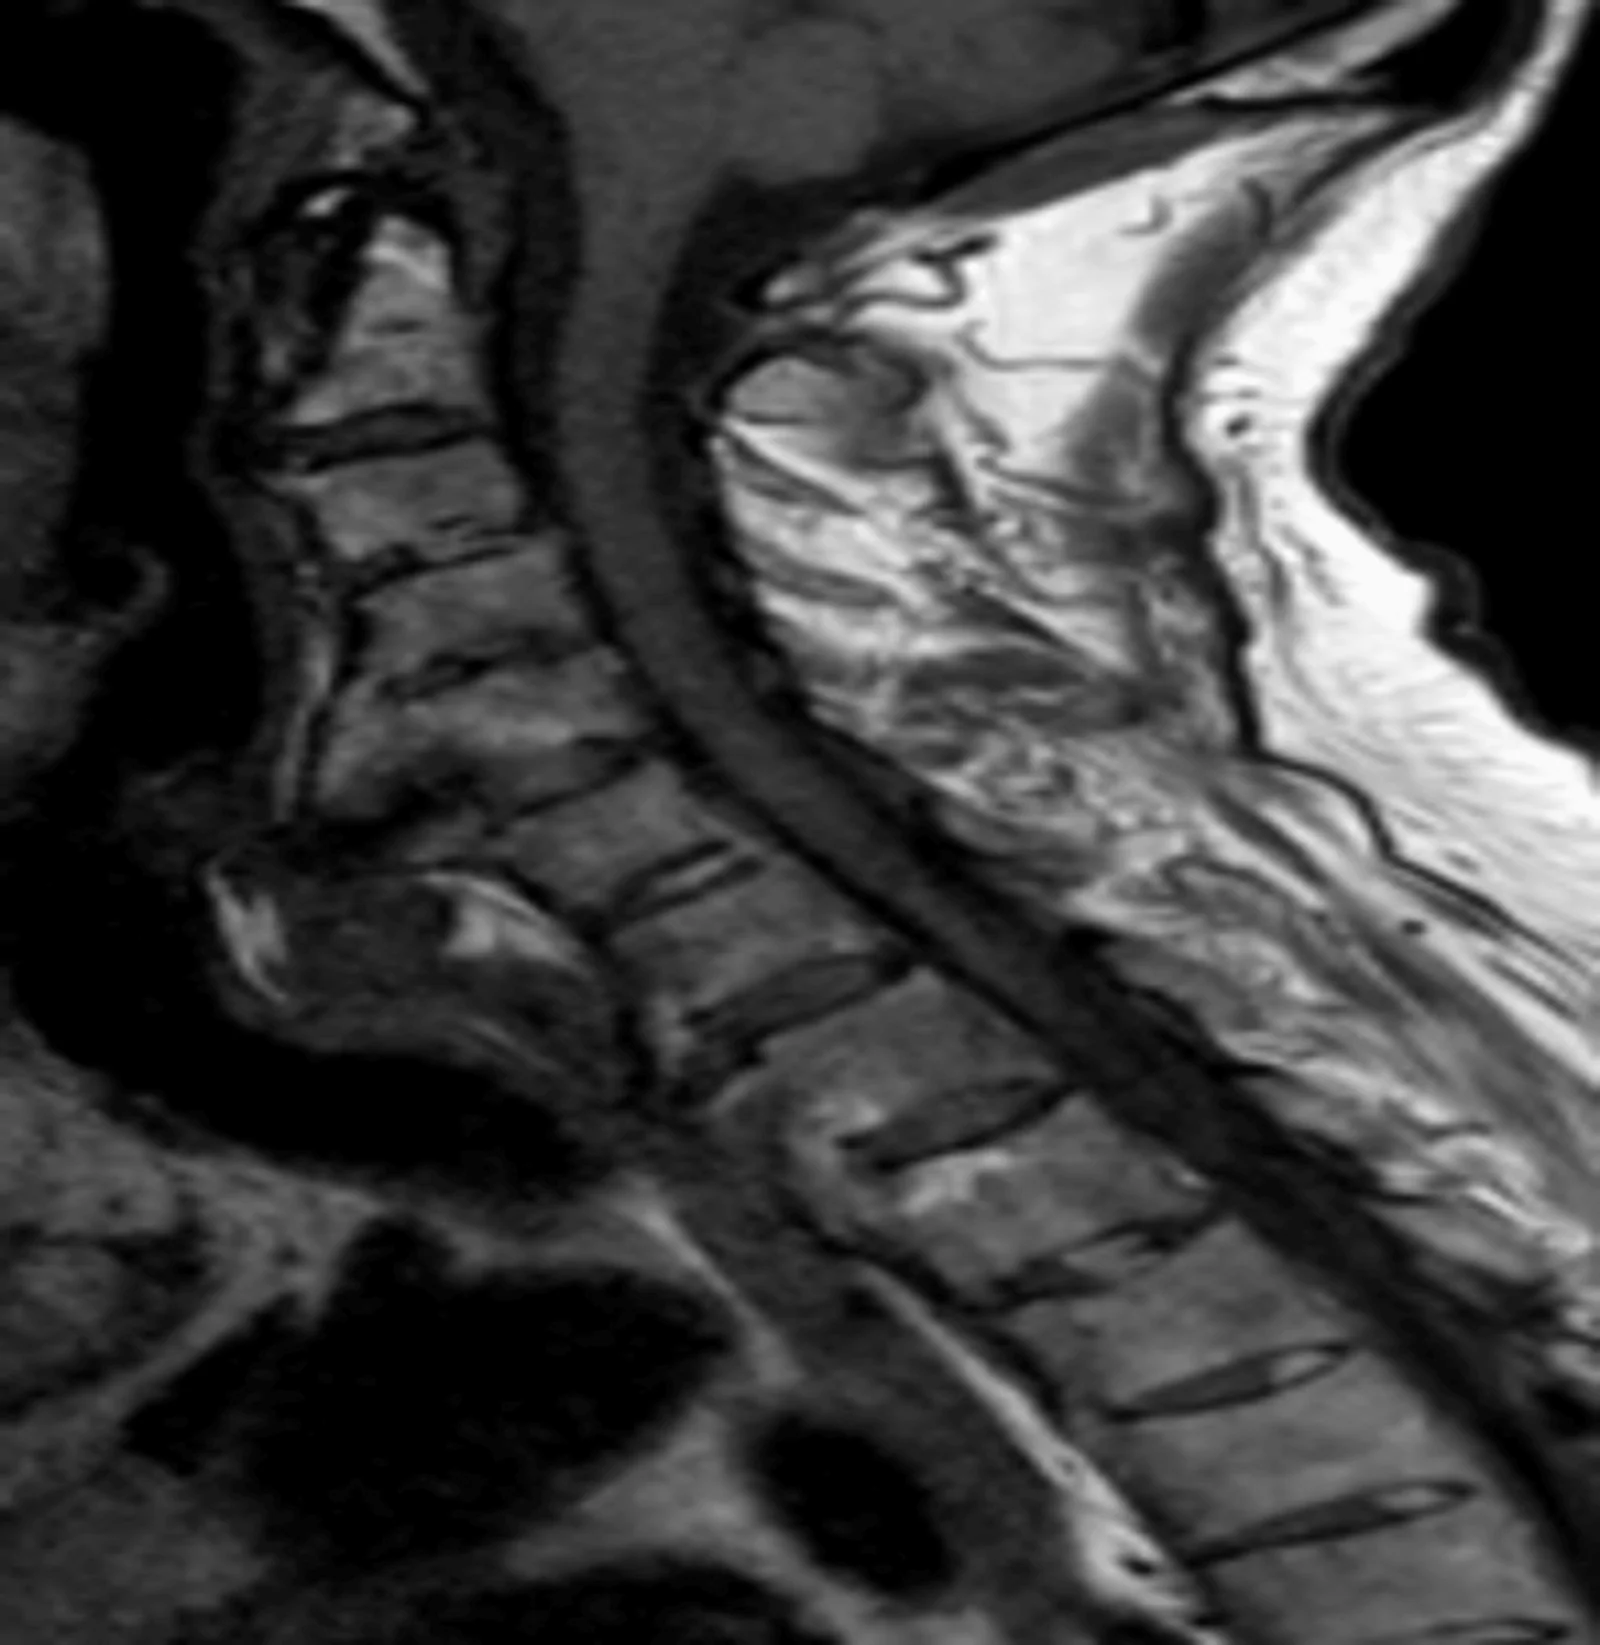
Magnetic Resonance Imaging (MRI) Sagittal cervical MRI demonstrating ossification of the anterior longitudinal ligament with bridging ossification at C3-C4 and C4-C5. Prominent right anterolateral marginal osteophytes are identified at C5-C6 and C7-T1, consistent with diffuse idiopathic skeletal hyperostosis (DISH).
